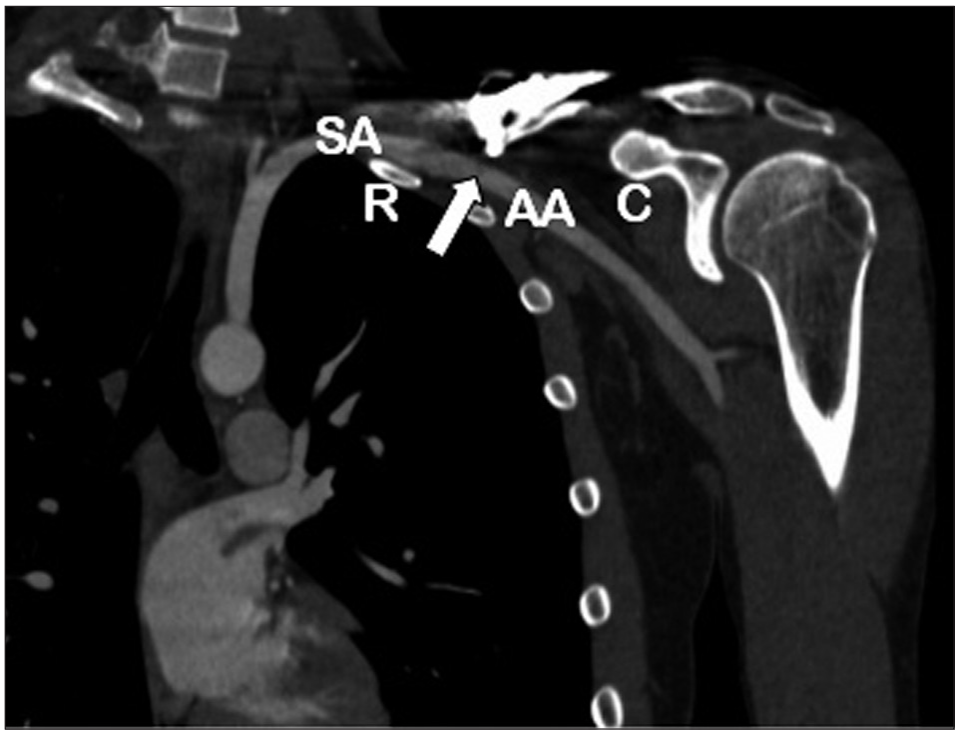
Coronal 2D reconstruction CT arteriogram of left shoulder. (AA: axillary artery; C: Coracoid process; R: 1^st^ rib; SA: Subclavian artery). Arrow depicts prominent screw with associated pseudoaneurysm. The subclavian artery becomes the axillary artery at the lateral border of the first rib. The subclavian artery lies in a groove on the superior aspect of the 1^st^ rib
